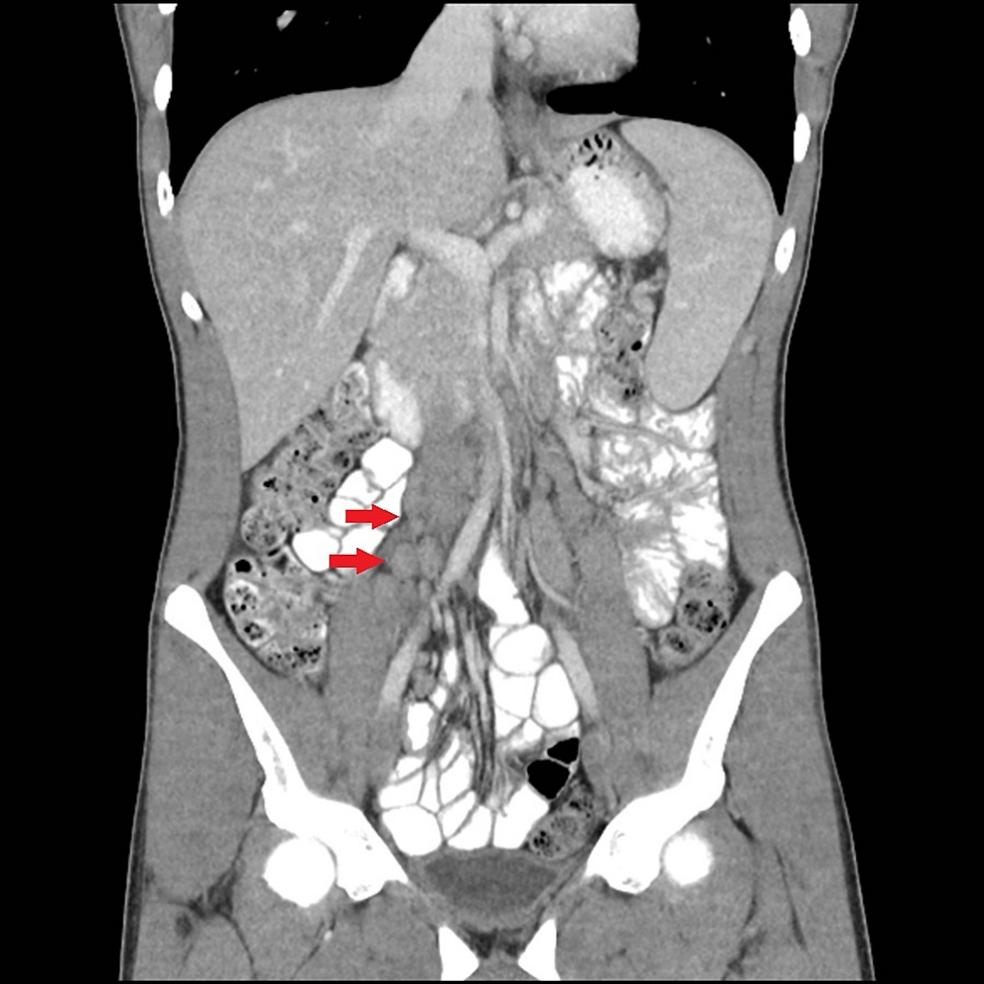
Coronal reconstructed image from CT abdomen and pelvis performed seven weeks after the initial CT shows stable enlarged mesenteric lymph nodes in the right lower quadrant (red arrows).
